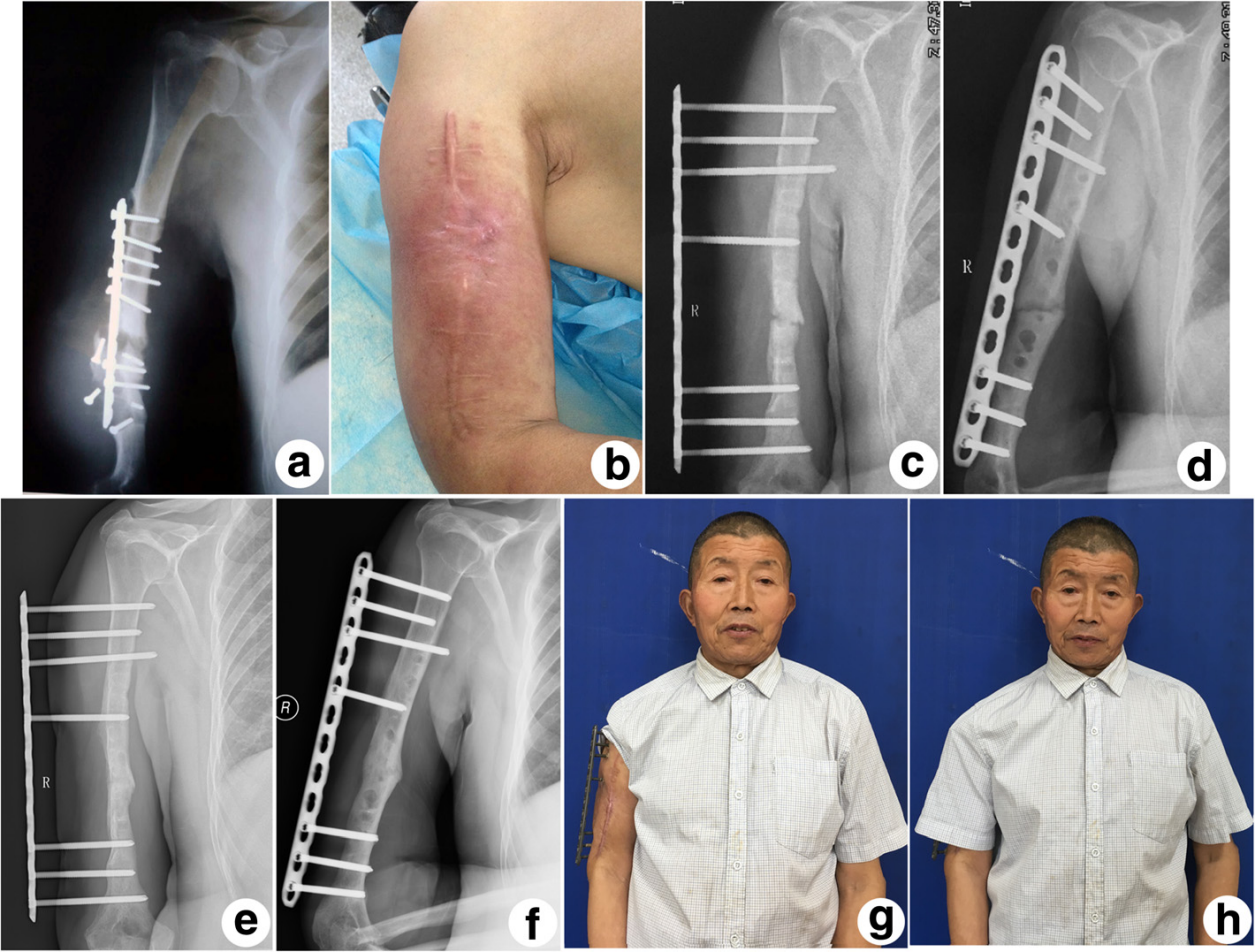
(a) X-ray of A 61-year-old male showing implant failure, nonunion and soft tissue swelling at the 15-month after the first surgery. (b) Extensive soft tissue swelling and erythema occurred in the affected upper limb. (c) Anteroposterior and (d) lateral X-ray at one month after revision surgery. (e) Anteroposterior and (f) lateral X-ray at the 6-month follow-up showing bony healing without any implant failure. (g) and (h) In situ plate showing low profile and well concealed under clothing
